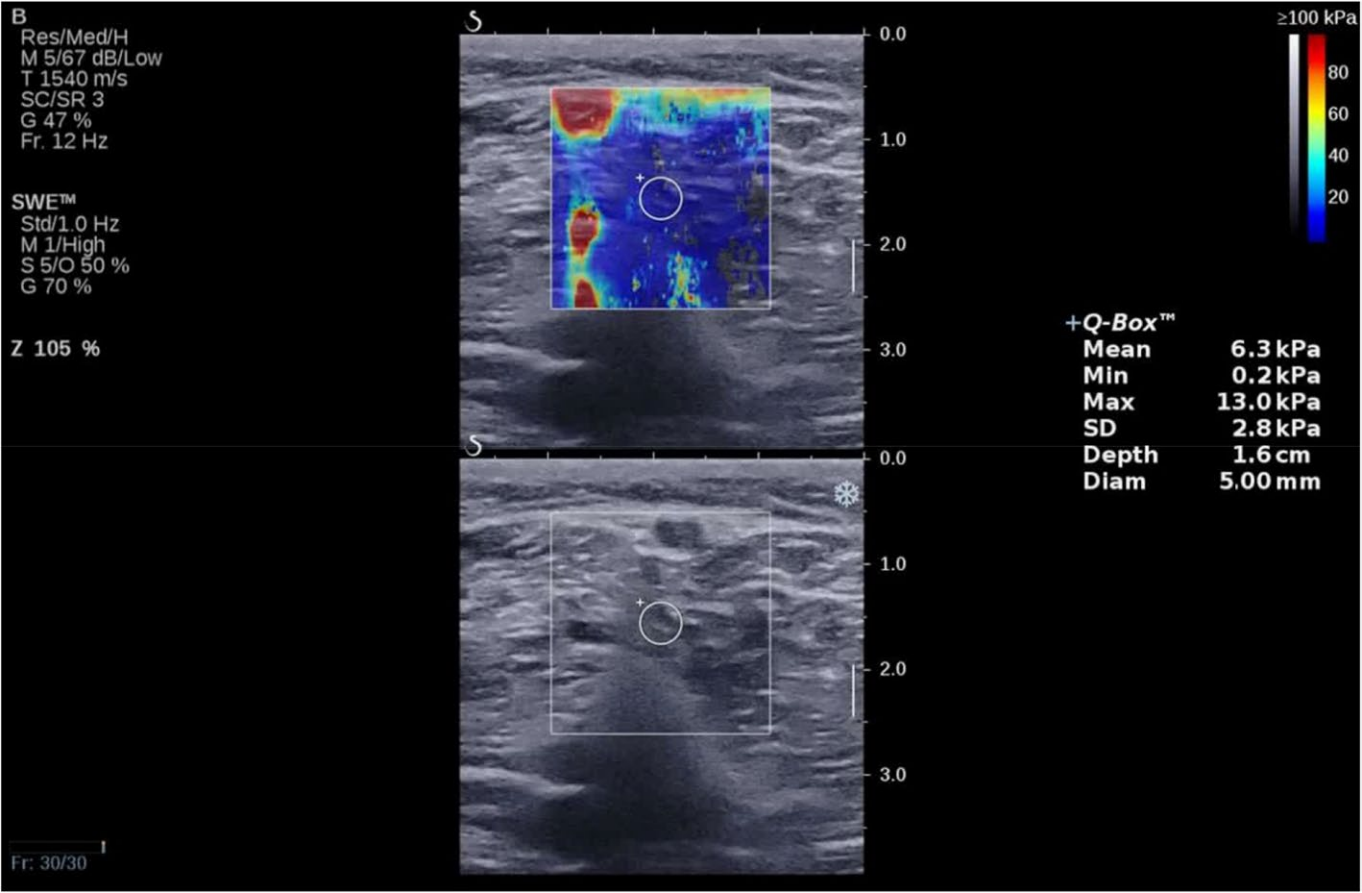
Typical elastography maps of the popliteus muscles. The upper images display color-coded boxes representing muscle stiffness. The bottom images show grayscale sonograms of the muscle. The measurement of shear modulus is conducted within a circular area, and the Q-Box is displayed on the right side for reference
